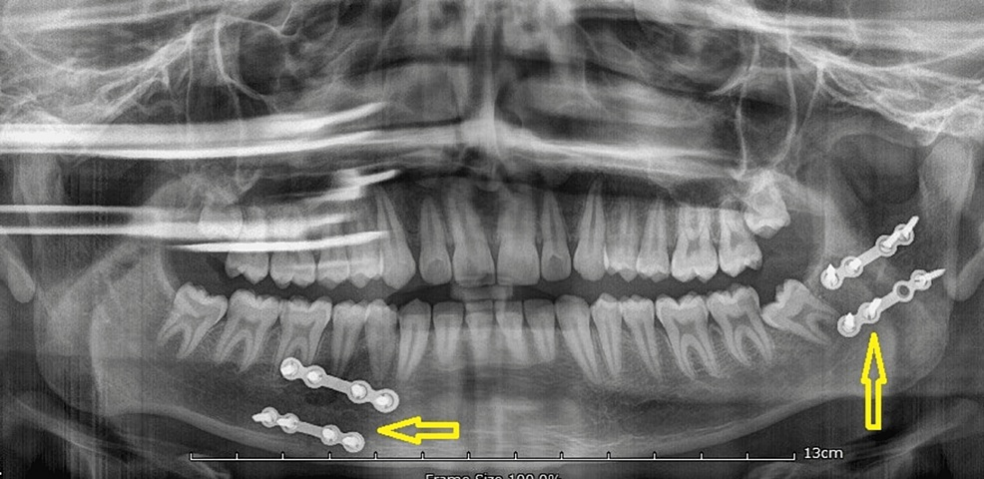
Postoperative OPG image Postoperative orthopantomogram (OPG) showing good wound healing (arrows)
